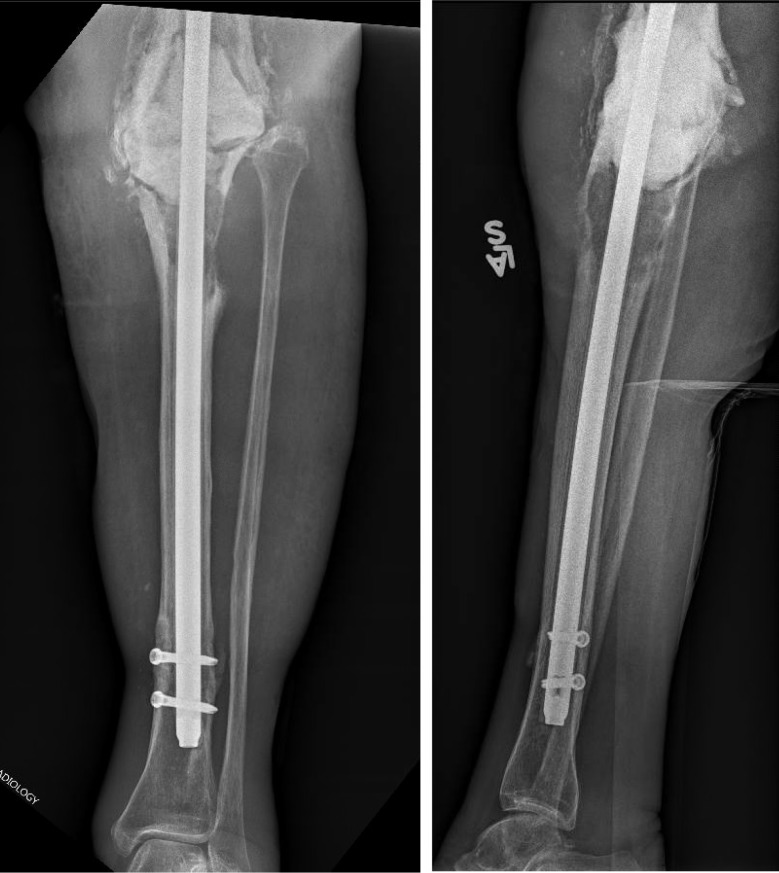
1/8/2018. AP (A) and lateral (B) radiographs demonstrating progressive lysis of the proximal tibia below the PMMA antibiotic cement spacer.
